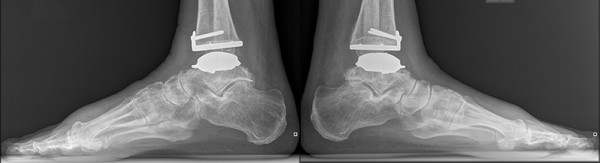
Lateral radiographs of both ankles 25 months after TAA. TAA centered correctly under the tibial axis.
